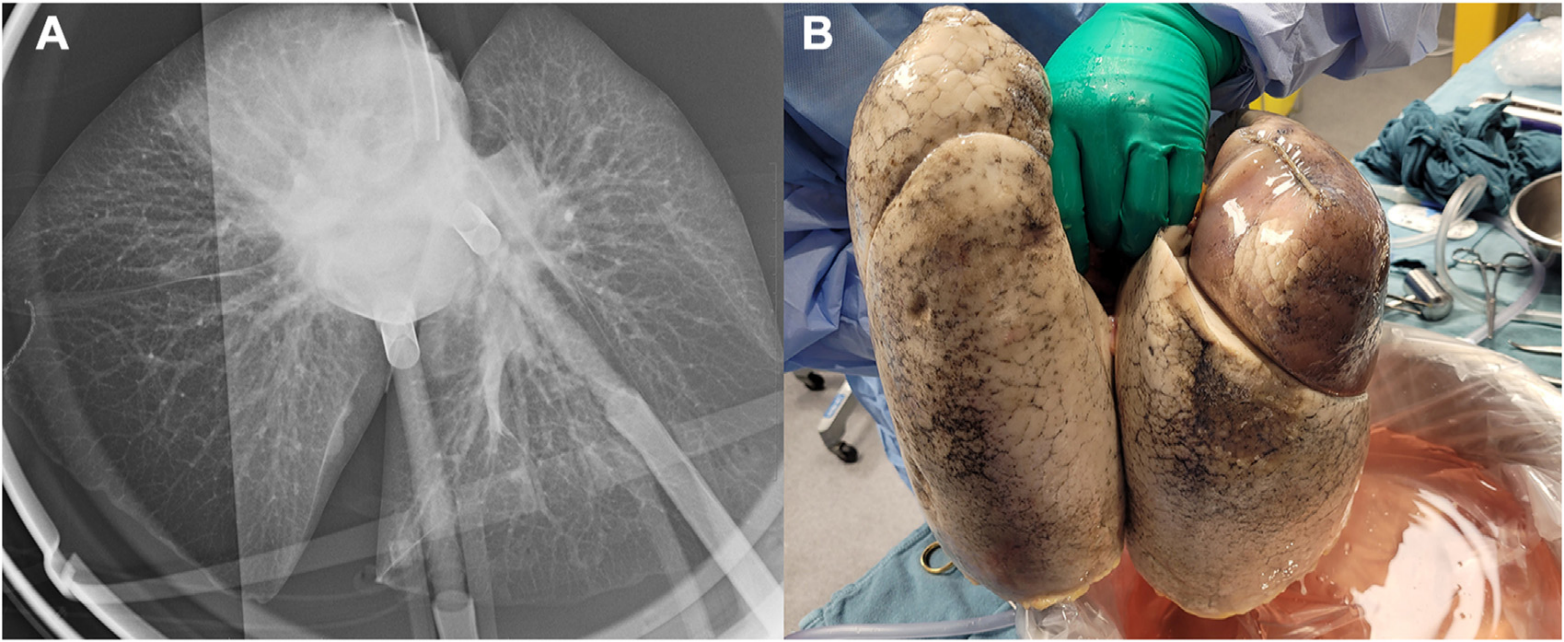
(A) Radiograph of ex vivo lung showing opacity in the right upper lobe. (B) Photo of donor lungs after ex vivo lung perfusion.
